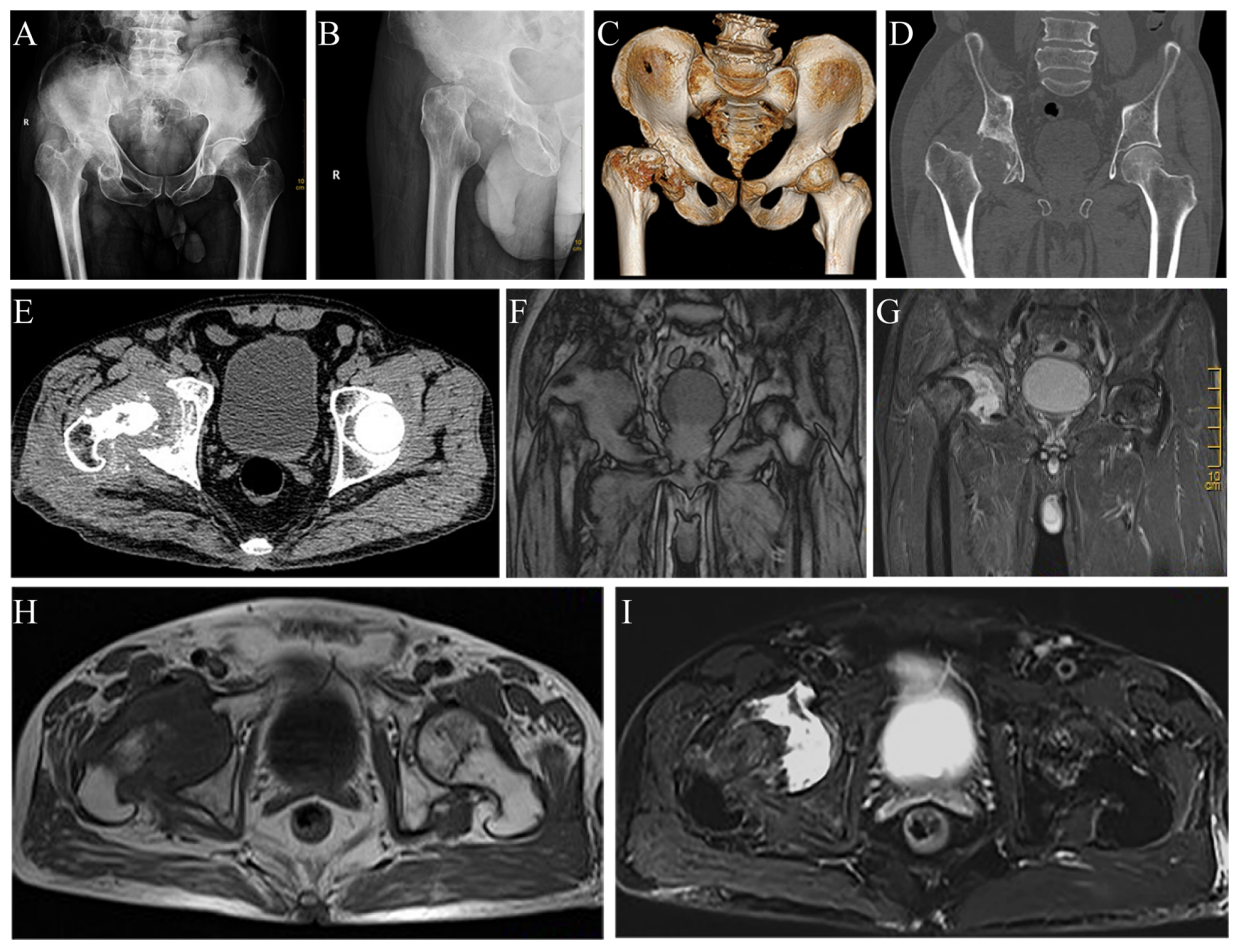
Radiograph images, 3D reconstruction image, CT scan images and MRI images of the hip joint revealing massive osteolysis in the right femoral head, femoral neck, and acetabulum. Anteroposterior (A) and lateral (B) radiographs of the hip joint show massive osteolysis in the right femoral head, femoral neck, and acetabulum. The 3D reconstruction image (C), coronal (D), and horizontal (E) CT scan images confirm the complete disappearance of the right femoral head and destruction of the femoral neck and acetabulum. Coronal (F) and horizontal (H) T1-weighted images show extensive hypointense osteolytic lesions involving the right femoral head, femoral neck, and acetabulum. Coronal (G) and horizontal (I) fat-suppressed T2-weighted images indicate extensive hyperintensity osteolytic lesions.
